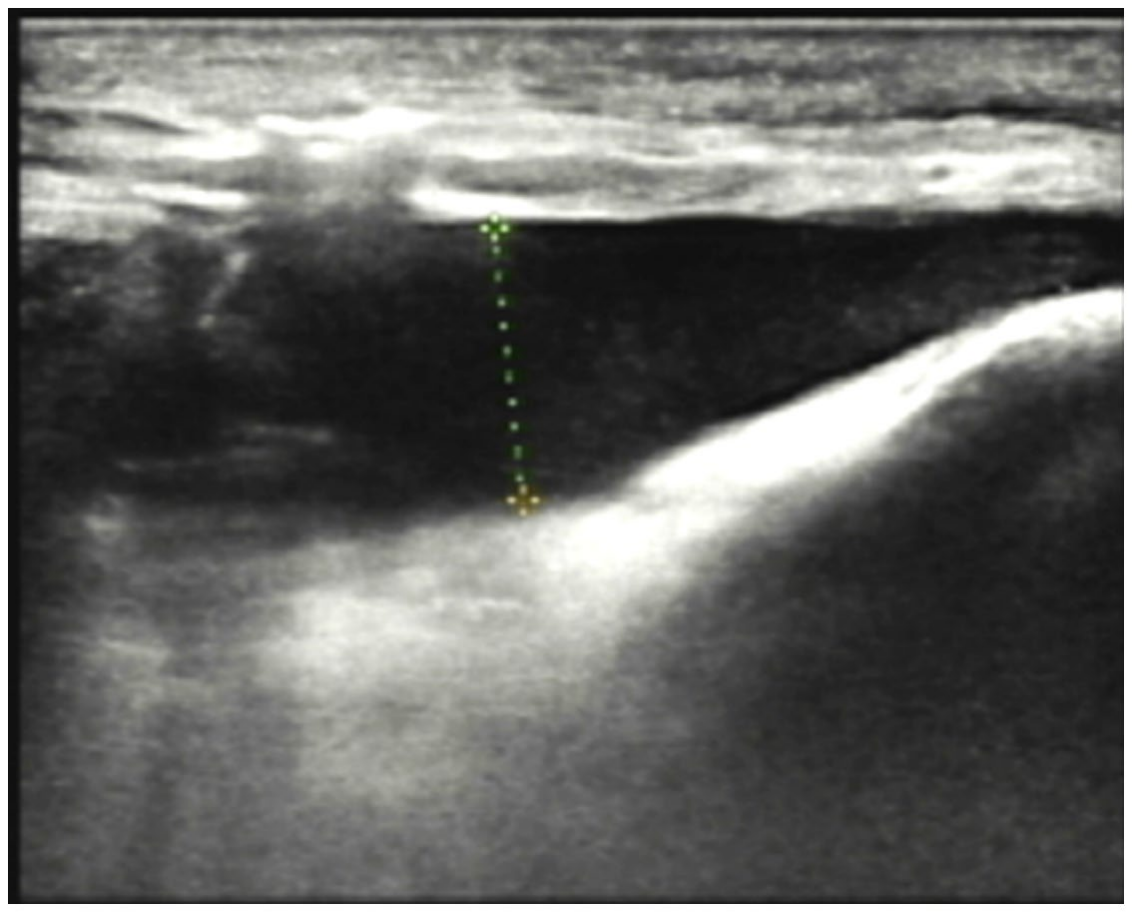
Ultrasound revealed subcutaneous soft tissue edema with a prominent dark fluid area.
